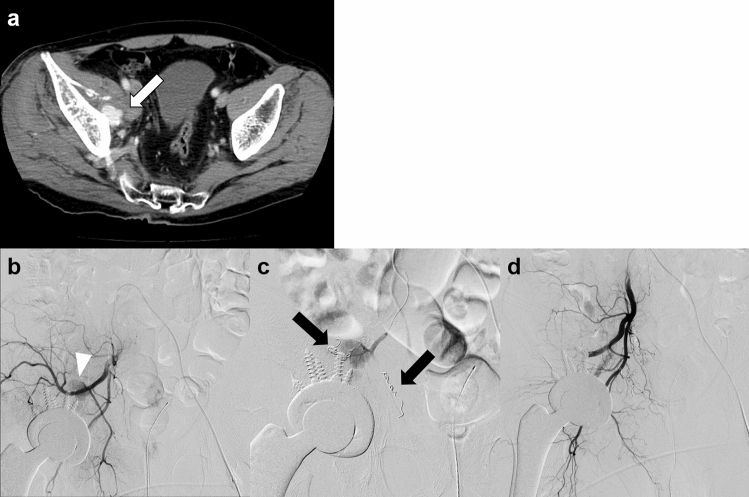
Pseudoaneurysm due to pelvic trauma in a 67 year-old man. a CT scan obtained 2 weeks after trauma shows pseudoaneurysm (arrow). b Digital subtraction angiography of the superior gluteal artery shows pseudoaneurysm (arrowhead). c Embolization of the obturator artery and distal portion of the superior gluteal artery has been performed with microcoils (black arrows) to avoid migration of NBCA to the peripheral arteries. d Selective embolization of the pseudoaneurysm with NBCA has been performed; digital subtraction angiography of the internal iliac artery shows the disappearance of the pseudoaneurysm. *CT* computed tomography; *NBCA*
